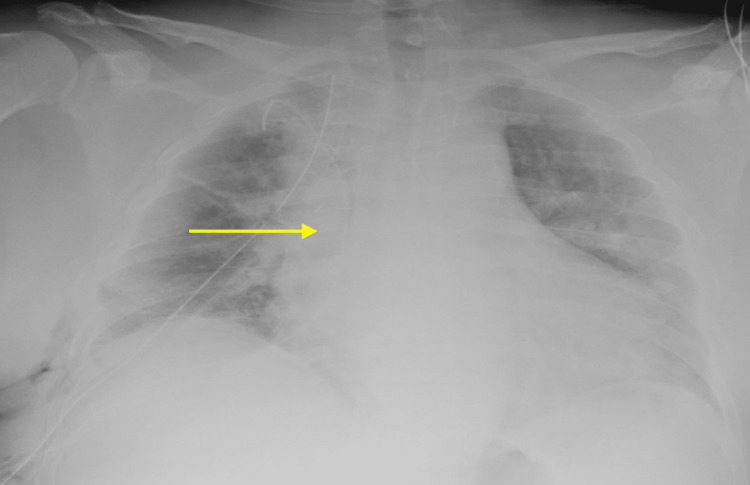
Chest X-ray (CXR) showing decreased right-sided consolidation and effusion (yellow arrow)
